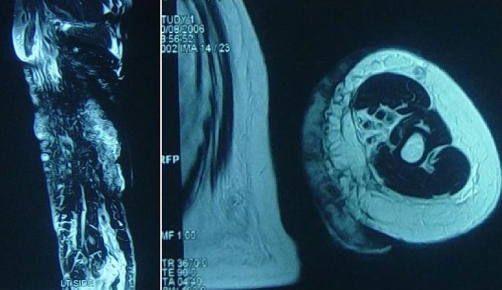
MRI of the left arm of the patient showing extension of the lesion marked with arrows.
